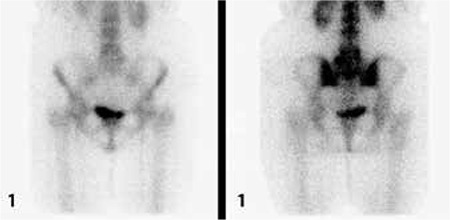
Tc-99m MDP bone scintigraphy images of a patient whose final diagnosis was degenerative changes however scintigraphy was reported as sacroiliitis and index values of the patient were also normal
